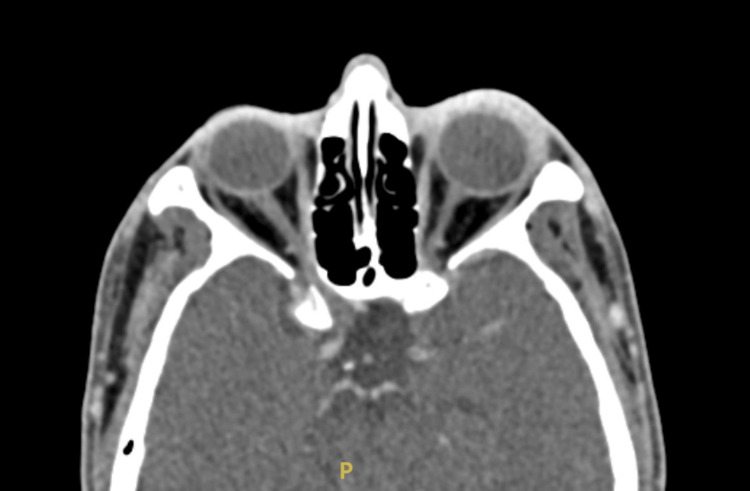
Axial computed tomography of the orbits with intravenous contrast demonstrating no intraocular or orbital foreign body and no evidence of post-septal involvement.
